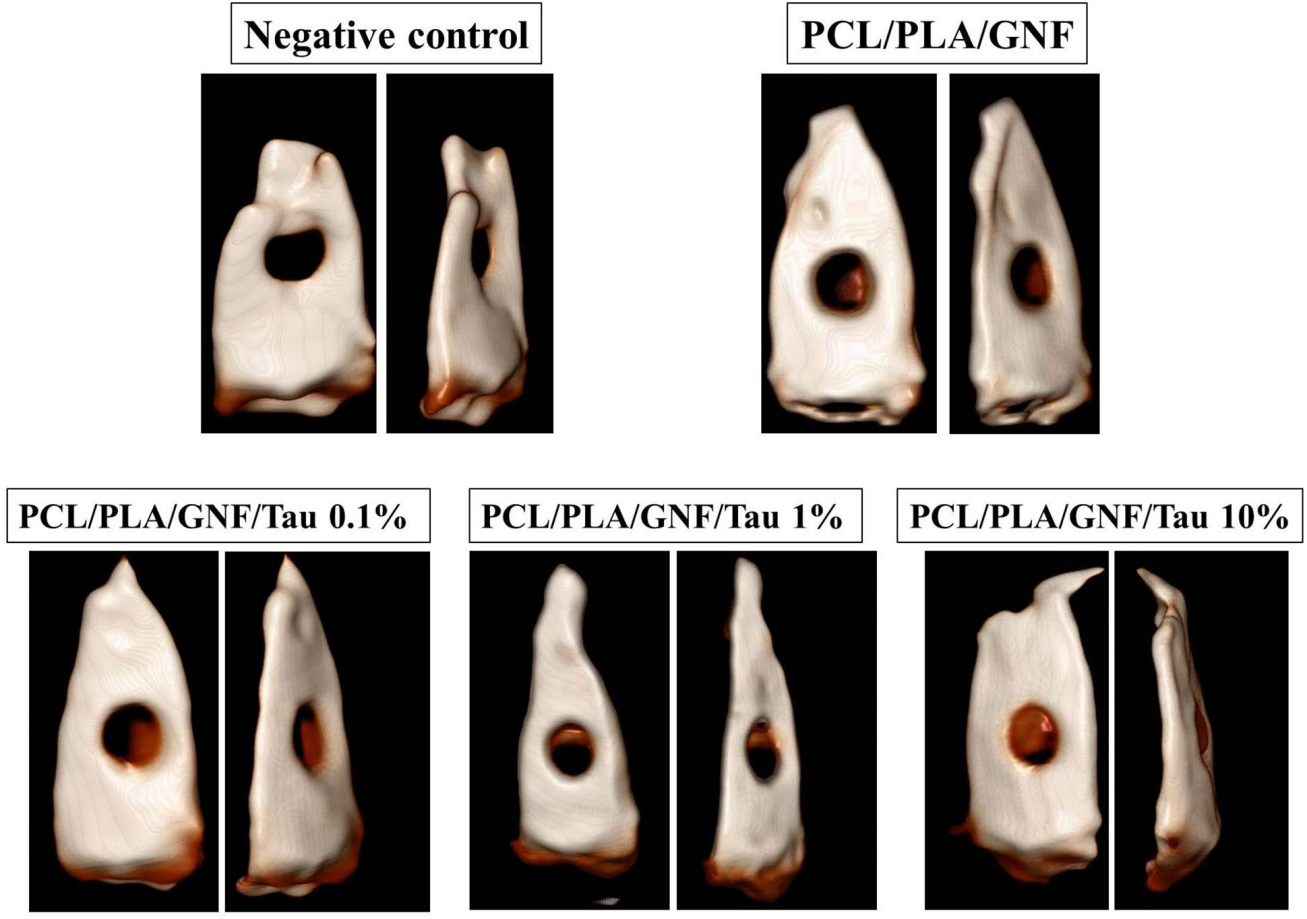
X-ray Computed Tomography (CT) images of damage skull bone. The images were obtained 12 weeks post-implantation.
